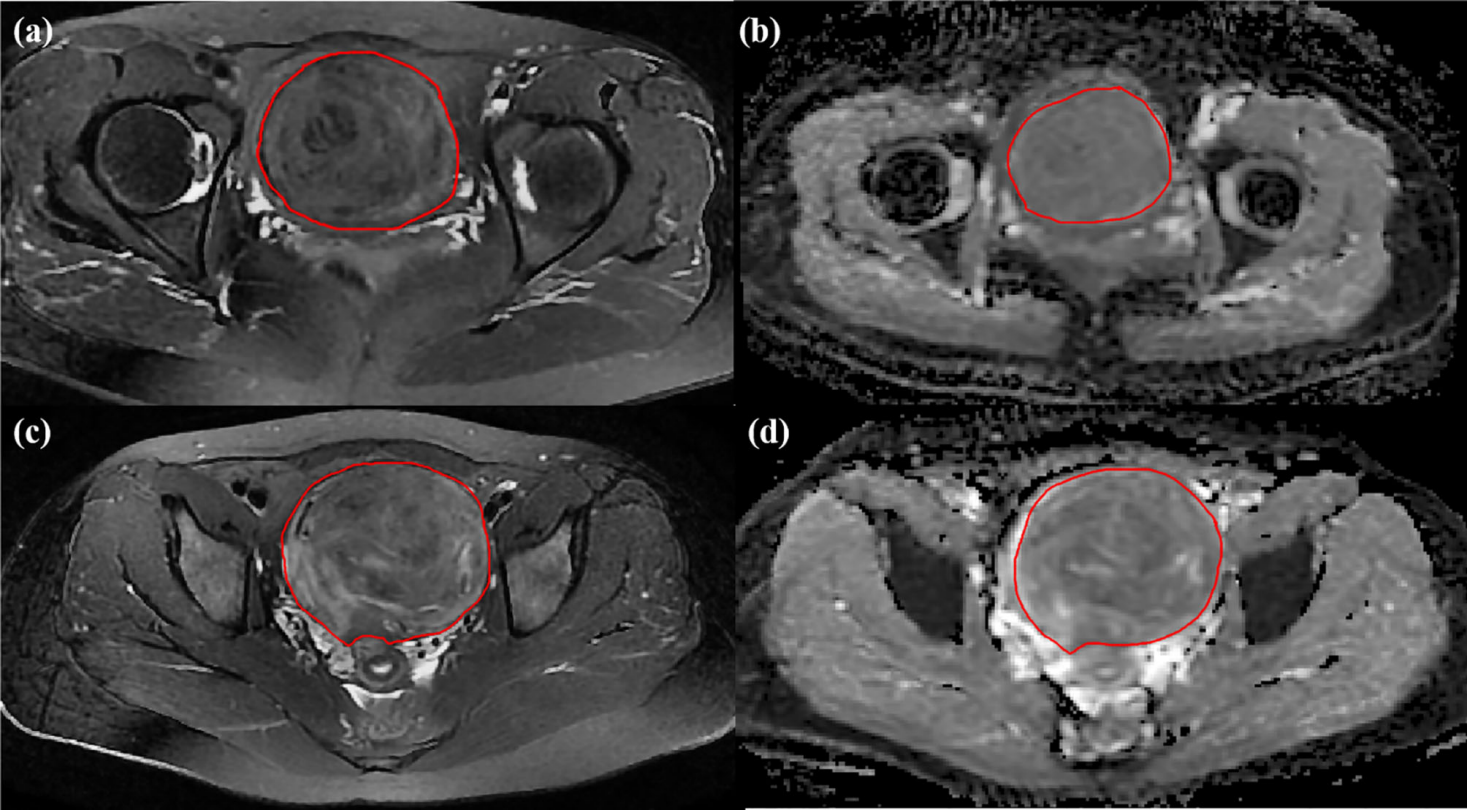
Two cases for delineating ROI. The preoperative MR images for uterine leiomyomas with sufficient ablation on (A) T2WI image and (B) ADC map from DWI and with nonsufficient ablation on (C) T2WI image and (D) ADC map from DWI.
